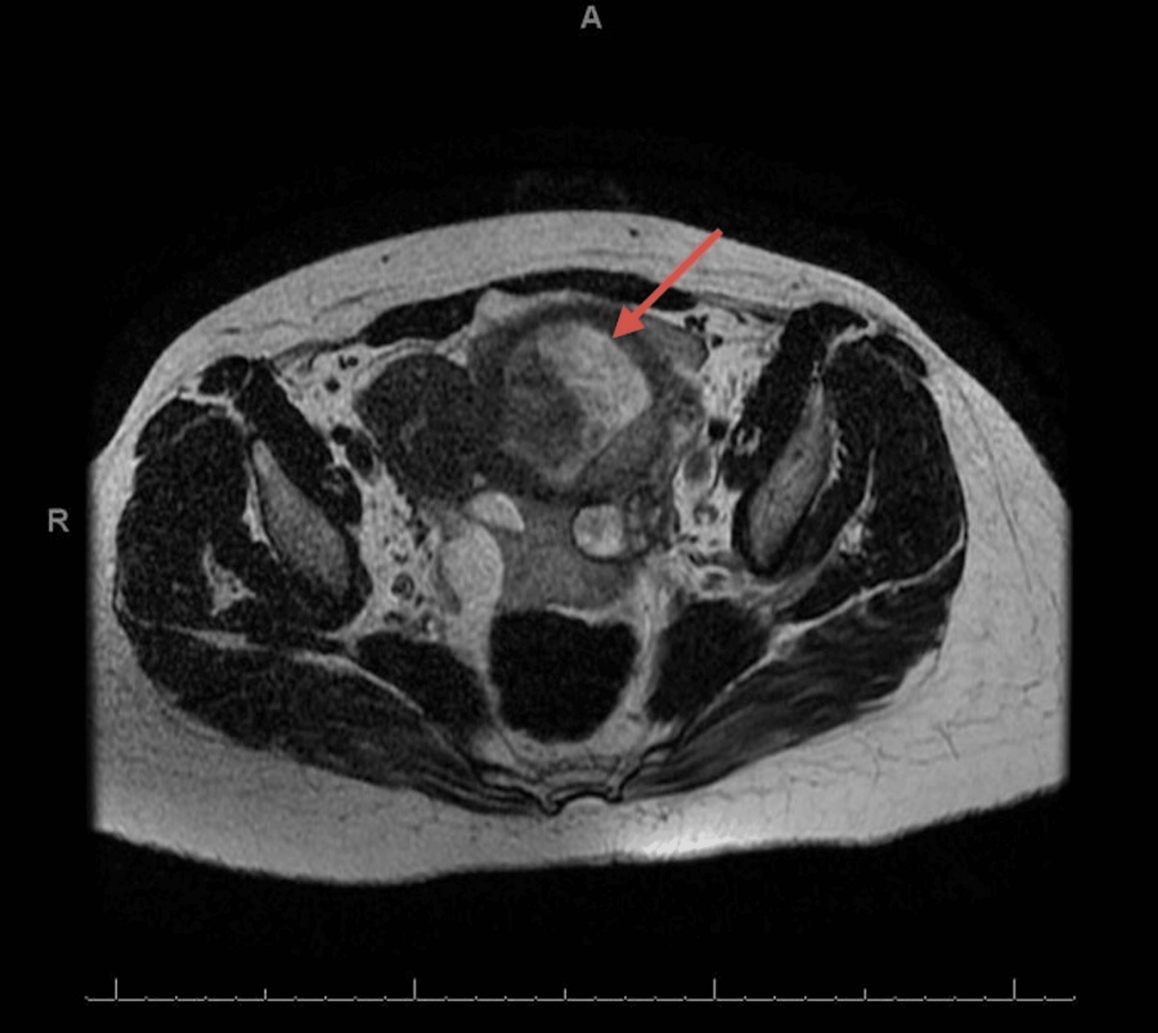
Axial image of MRI showing uterus and fetus inside the rudimentary horn (red arrow).
